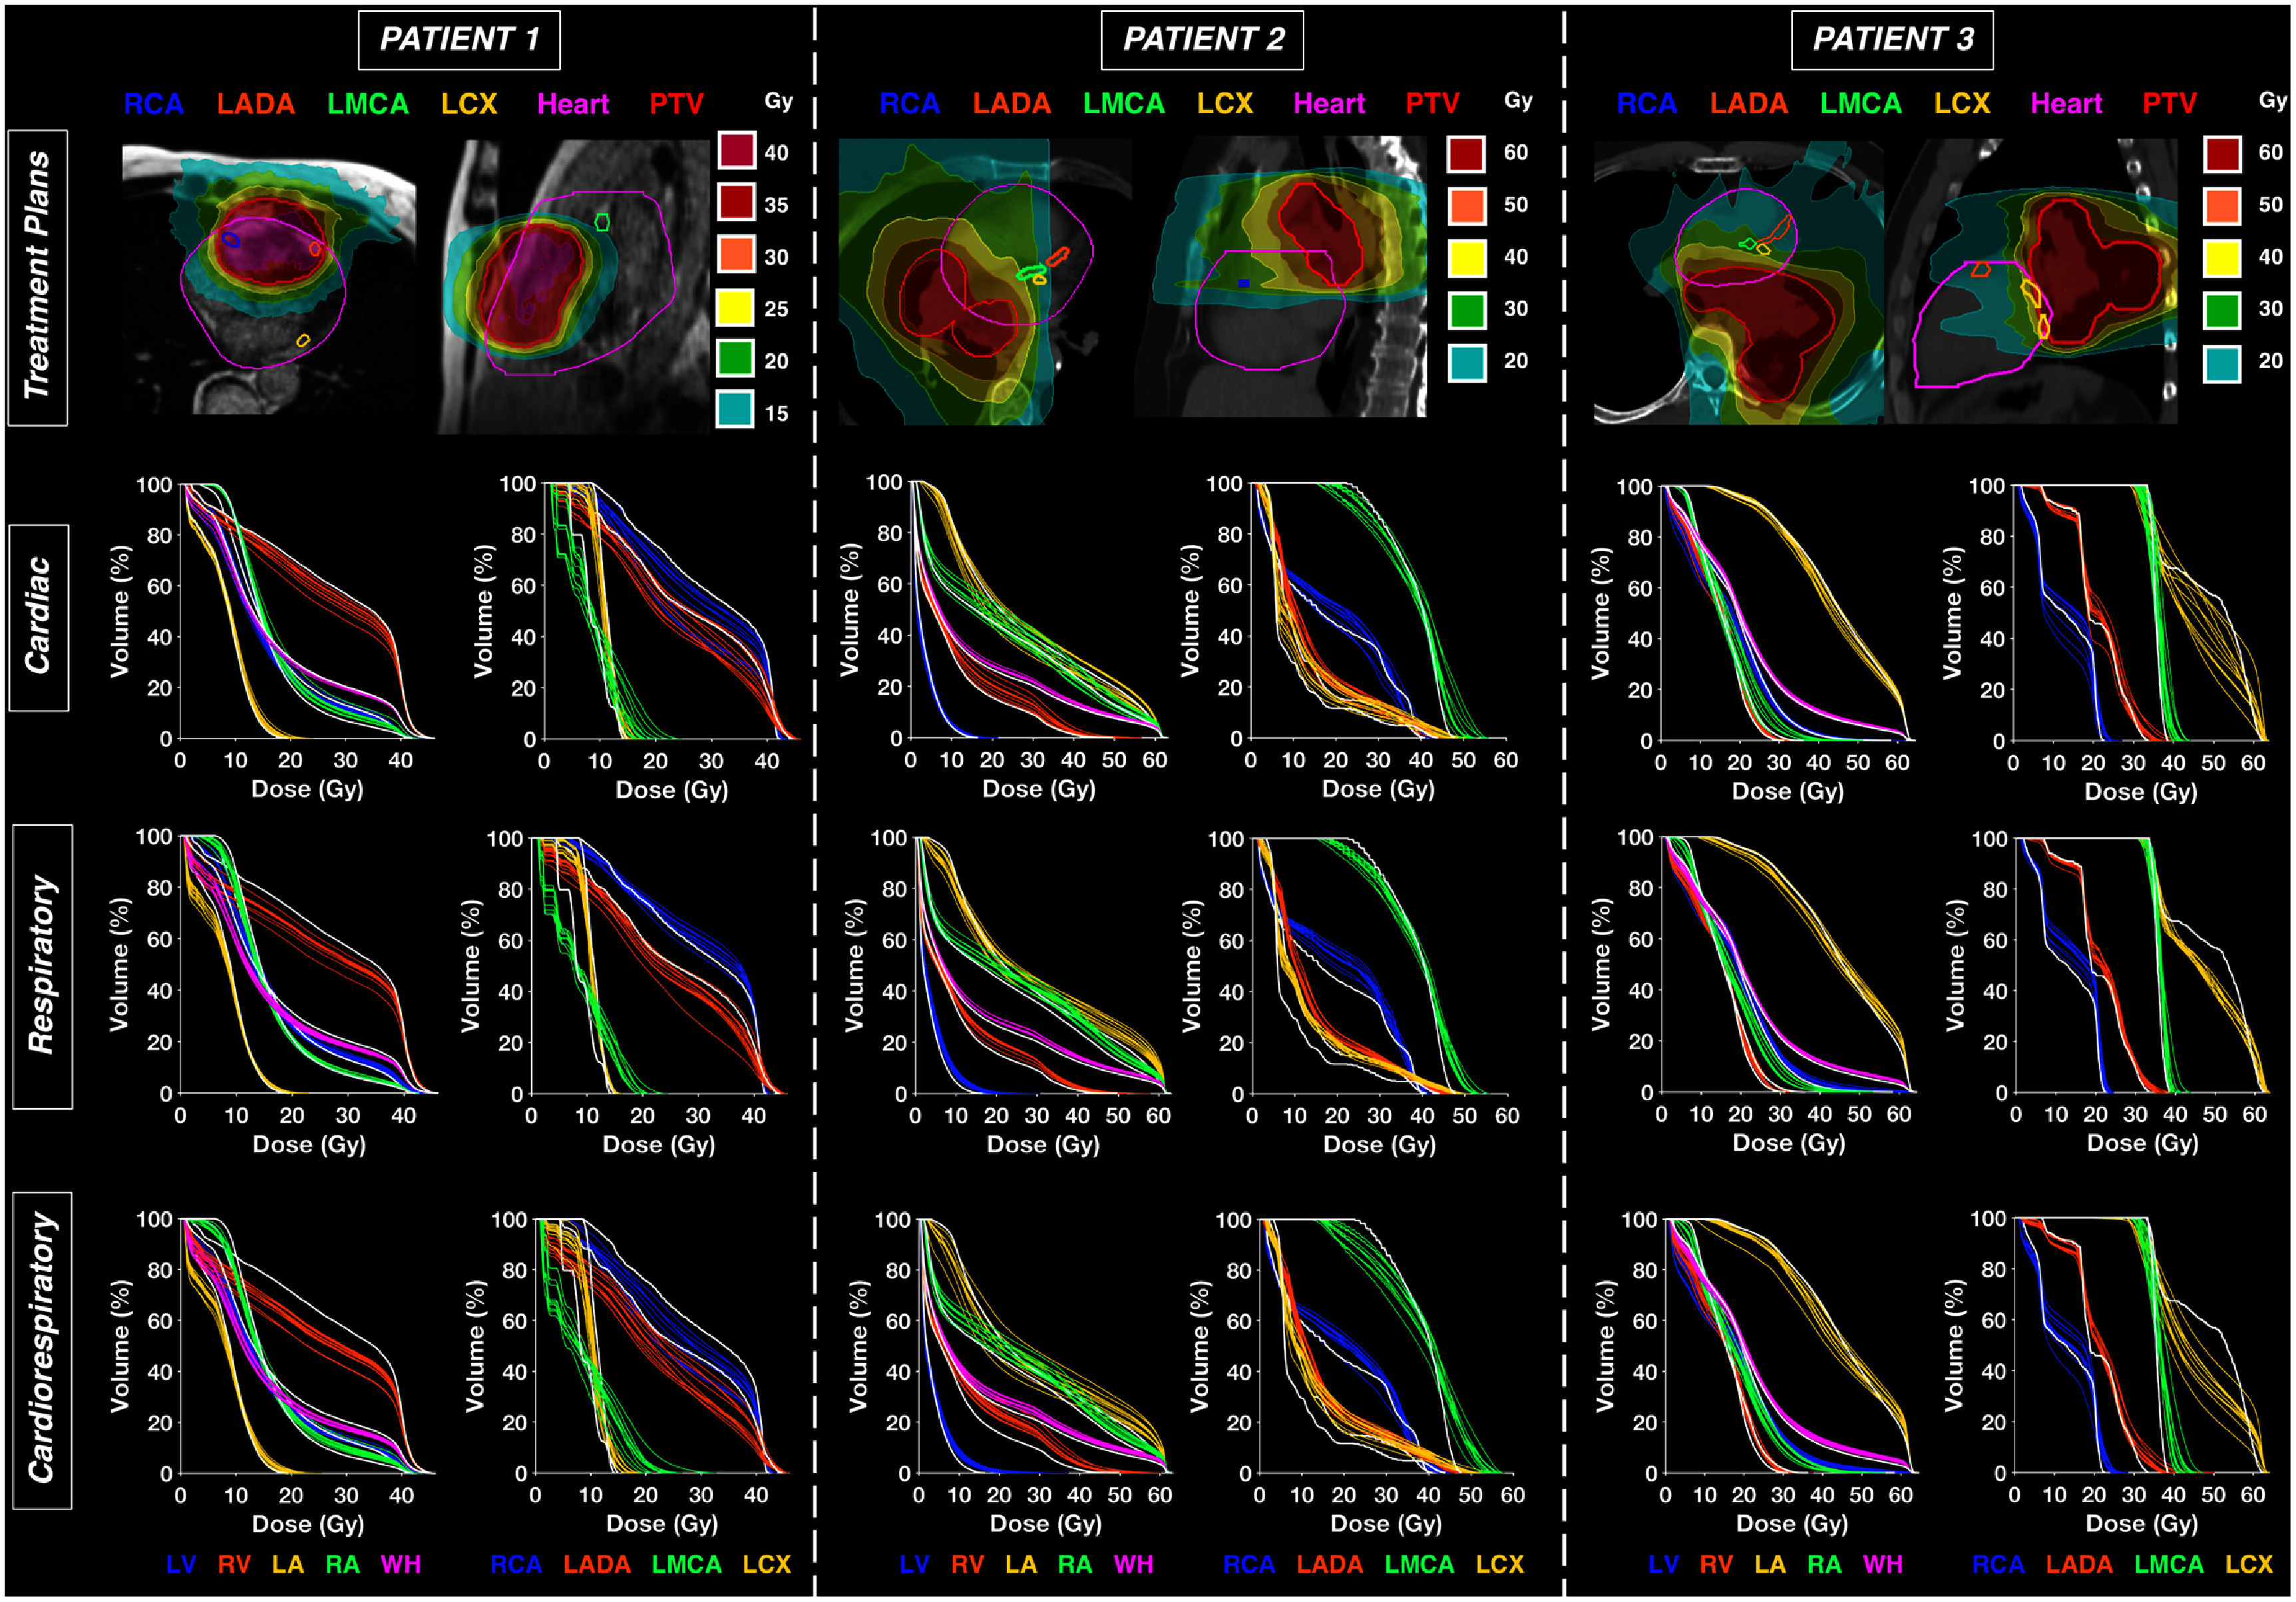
Dosimetric analysis for 5D-MRI-derived internal organ at risk volumes (IRVs) for three patients (top) with (left) a metastasis to the anterior region of the heart, (middle) right-middle lobe lung cancer, and (right) left-lower lobe lung cancer. Dose volume histograms (DVHs, bottom) summarize doses for the coronary arteries and chambers for each patient treatment plan. DVHs for cardiac substructures without added IRVs are shown with a white line, and each individual colored line corresponds to a 5D-MRI-derived IRV from our cohort of 10 volunteers. Isolated cardiac (top), isolated respiratory (middle), and combined cardiorespiratory (bottom) IRVs are shown.
